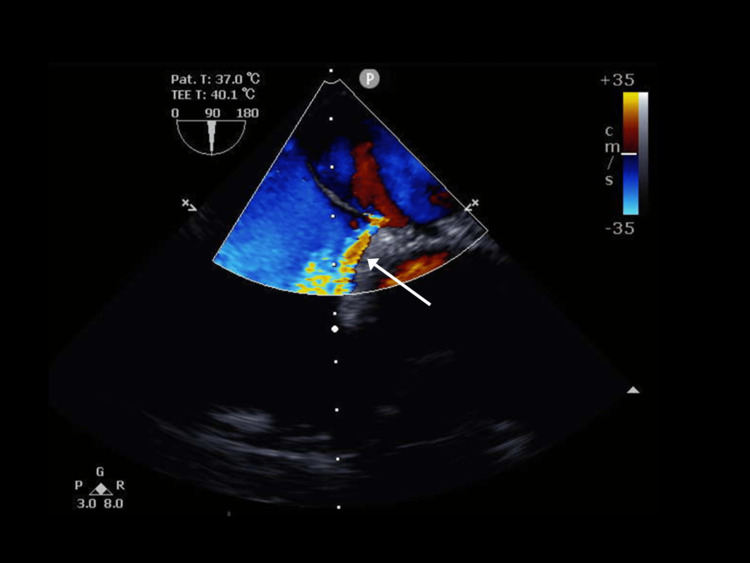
Transesophageal echocardiography shows blood flow from the left atrium to the right atrium at rest through the foramen ovale (arrow).
